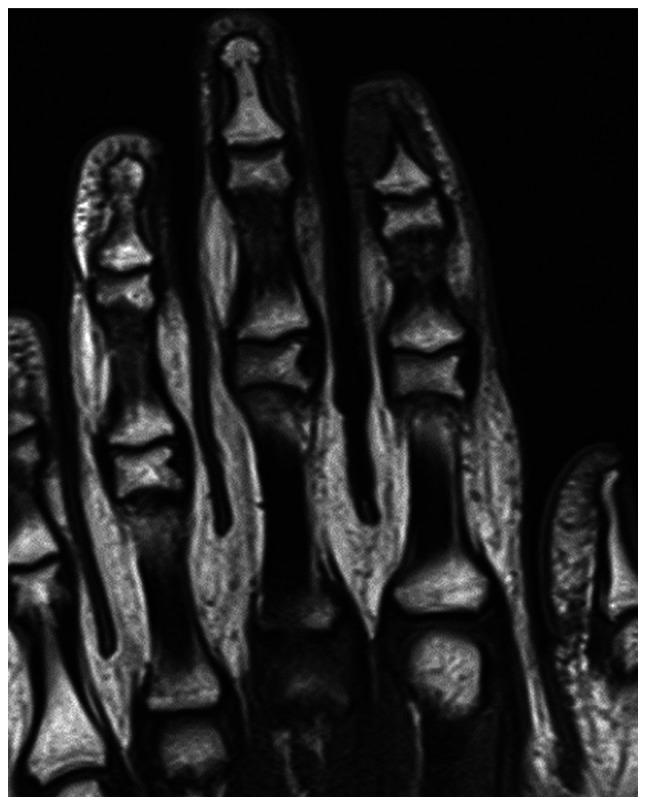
Coronal T1-weighted magnetic resonance imaging scan of the lesion in the right index finger.
